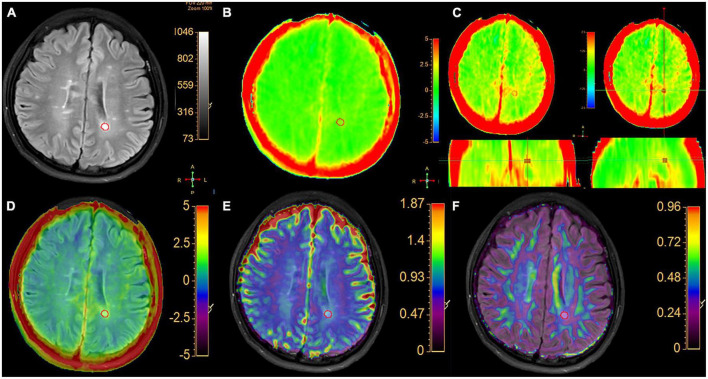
A typical MS patient. (A) The FLAIR image and the hyperintense area in white matter were segment semi-automatically delineated regions of interest. (B) APTw image with –5 to 5% display range. (C) APTw images of three planes with a –2.5 to 2.5% display range. The VOI of lesion is shown in three planes with selected MTRasym (3.5 ppm) values. (D) APT and FLAIR image fusion. (E) ADC and FLAIR image fusion. (F) FA image fused with FLAIR image.
